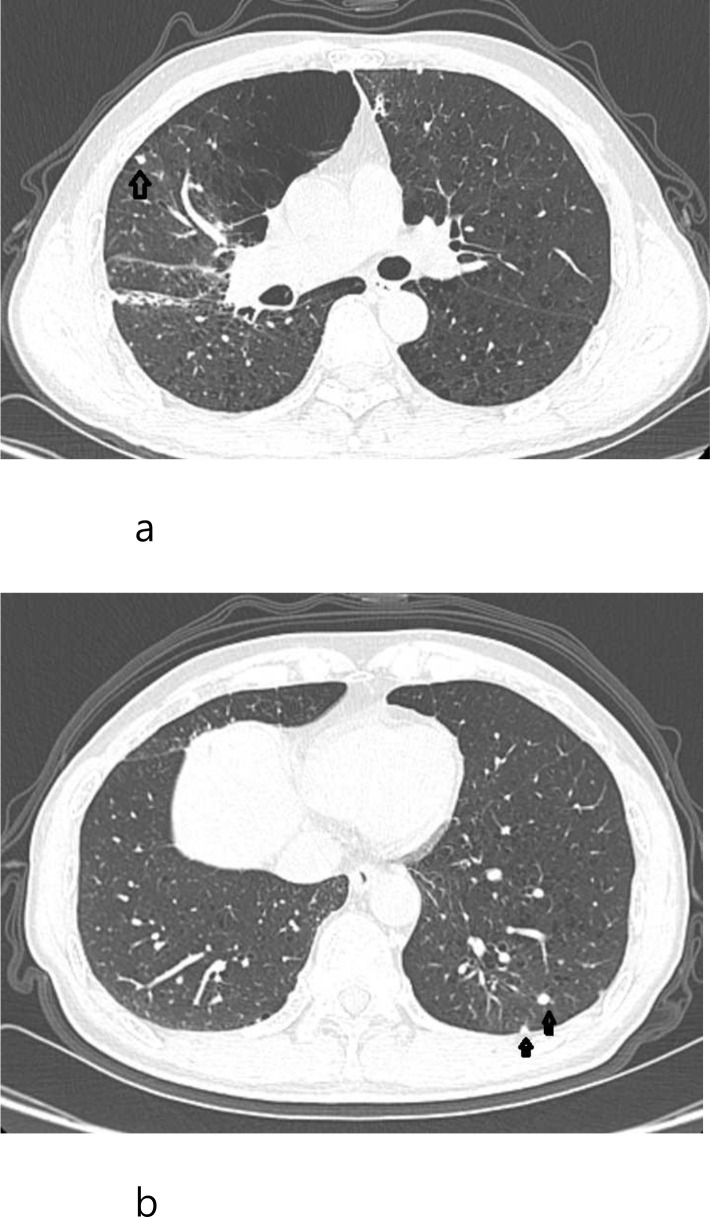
March 2026 CT follow-up scan of lung. (a) The original thyroid lesion shows no progression. (a) A nodule is visible in the right lung at the arrow. (b) Two nodules are visible in the left lung at the arrow.
